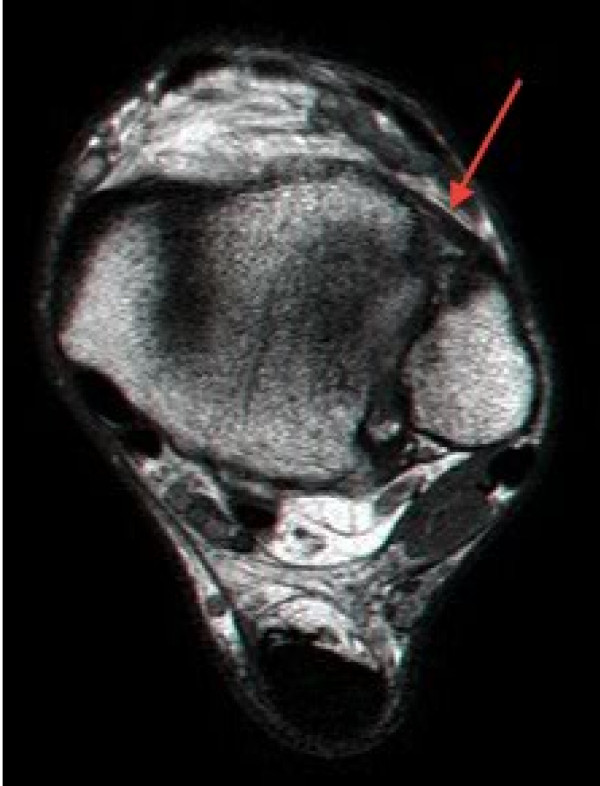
An axial T1 Turbo Spin Echo magnetic resonance (MR) image, above joint level, where ultrasound measurements are taken. Note the borders of the healthy Antero Inferior Tibio-Fibular Ligament (AITFL) marked with a red arrow.
